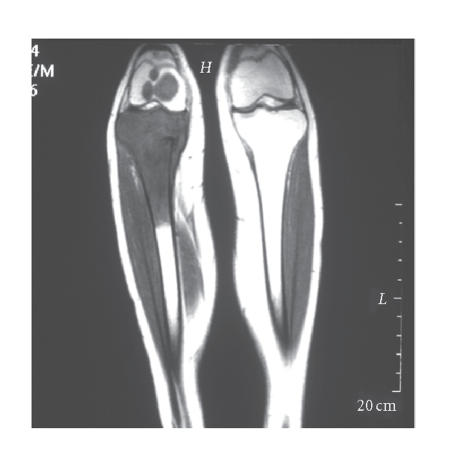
MRI right leg, showing osteosarcoma of proximal tibia
